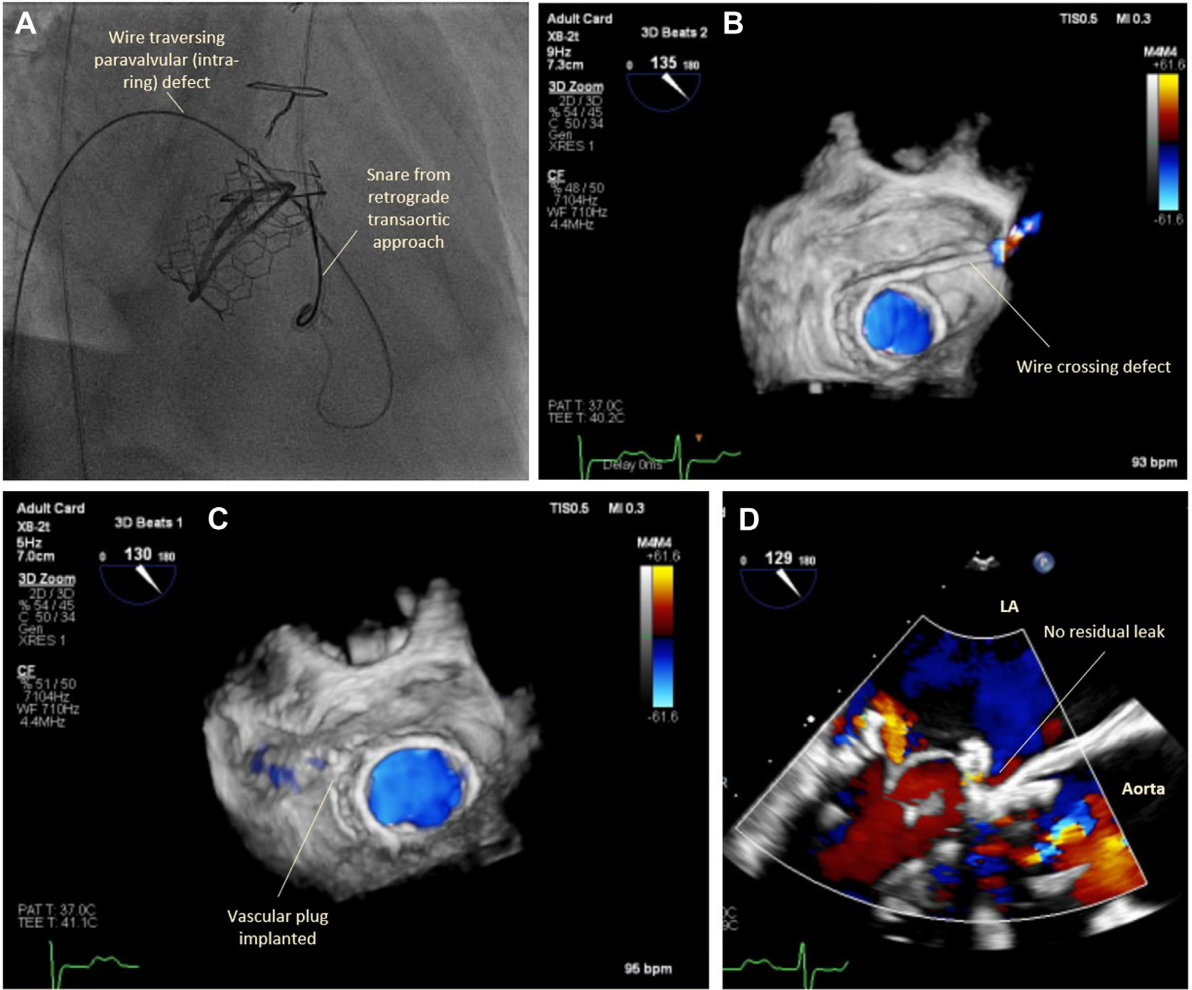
Closure of the Paravalvular Leak Closure of the paravalvular leak by (A and B) traversing the defect with a wire and (C) implantation of a vascular plug. (D) The final result on transesophageal echocardiography with color Doppler is shown, demonstrating no significant leak. LA = left atrium.
